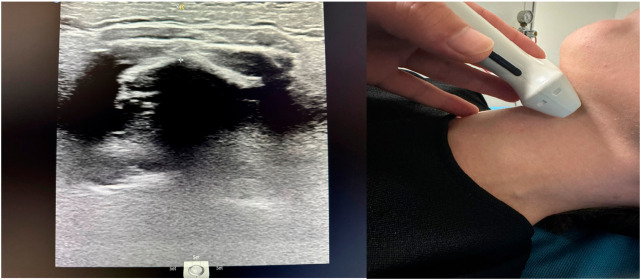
Ultrasonographic measurement of the skin-to-hyoid-bone distance (SHBD): linear probe placed transversely (right) and the corresponding sonographic view (left).
